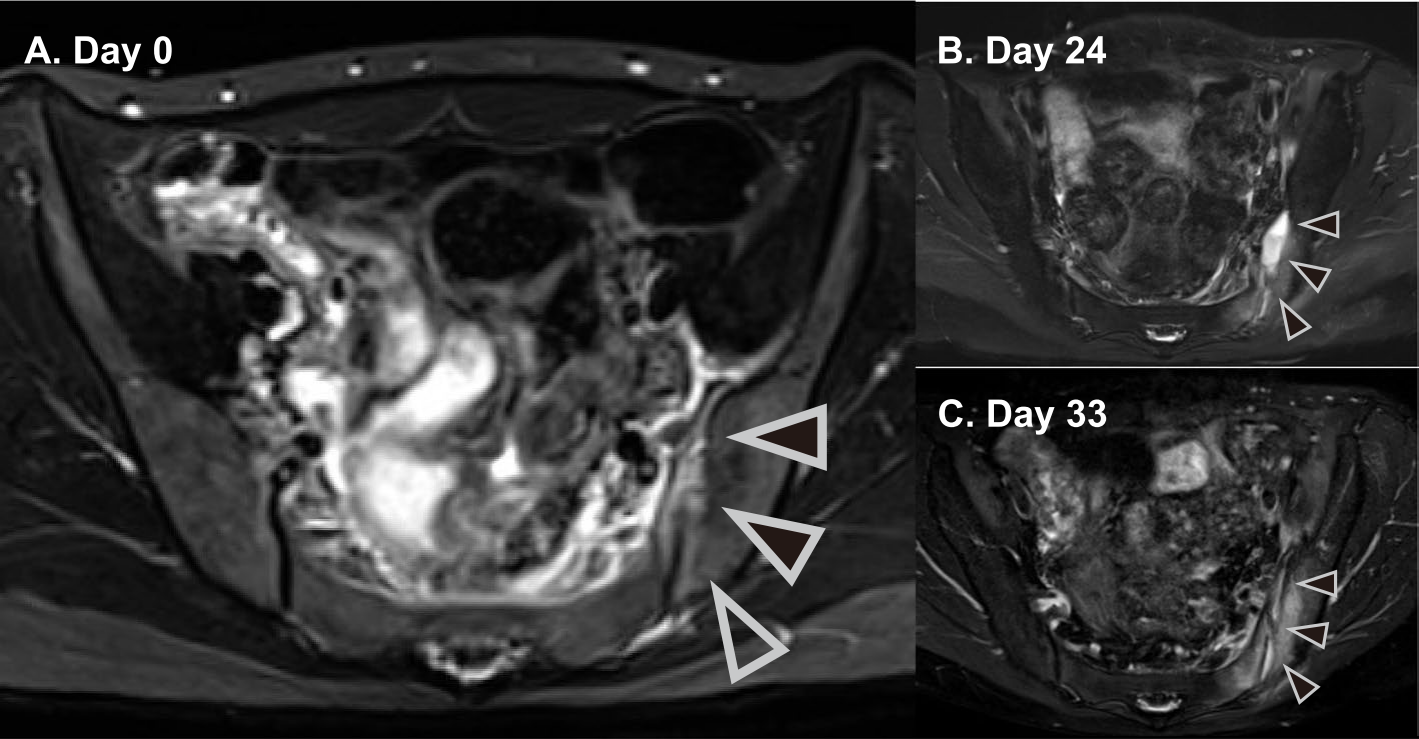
Pelvic magnetic resonance image (A) upon admission and days (B) 24 and (C) 33. The arrowhead indicates fluid collection at the left sacroiliac joint
